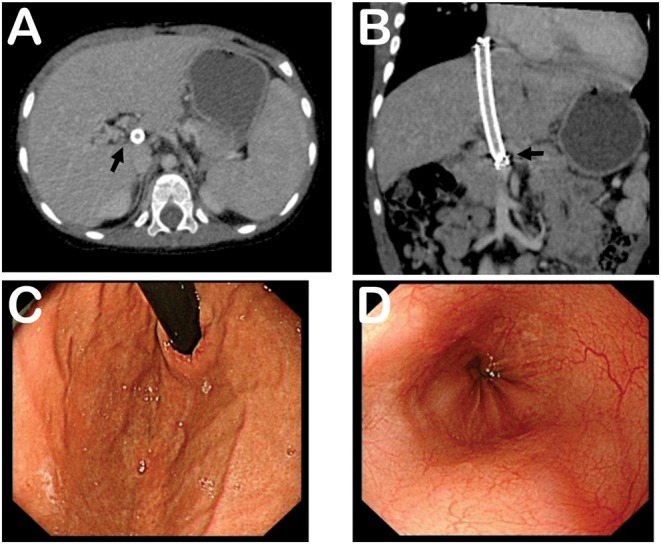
Follow-up computed tomography and esophagogastroduodenoscopy at 6 months post-TIPS. (A) Computed tomography showing shunt and smaller size of spleen. (B) Tomographic reconstruction showing stent and portal vein. (C) Esophagogastroduodenoscopy demonstrating relieved gastric varices. (D) Esophagogastroduodenoscopy revealing relieved esophageal varices. Black arrows indicate stent and portal vein.
